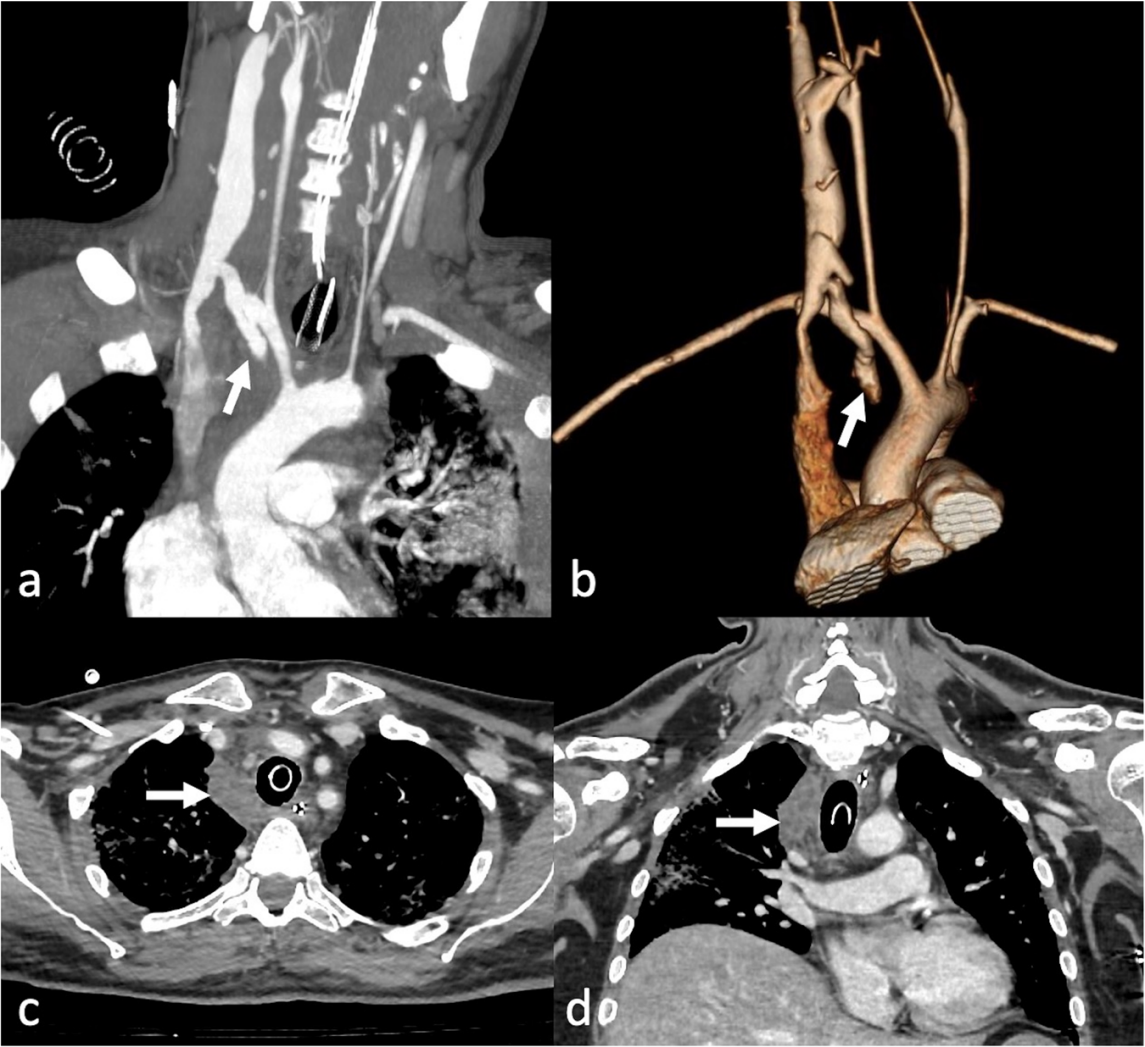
Coronal computed tomography (CT) images in the venous phase, with maximum intensity projection (MIP) reconstruction (a) and 3D volume rendering (3D-VR) reconstruction (b), show contrast medium extravasation (arrows) due to incorrect cannulation of the right jugular vein, causing vessel wall rupture. Axial (c) and coronal (d) CT images in the venous phase of the same patient demonstrate a blood collection in the right paratracheal space (arrow)
